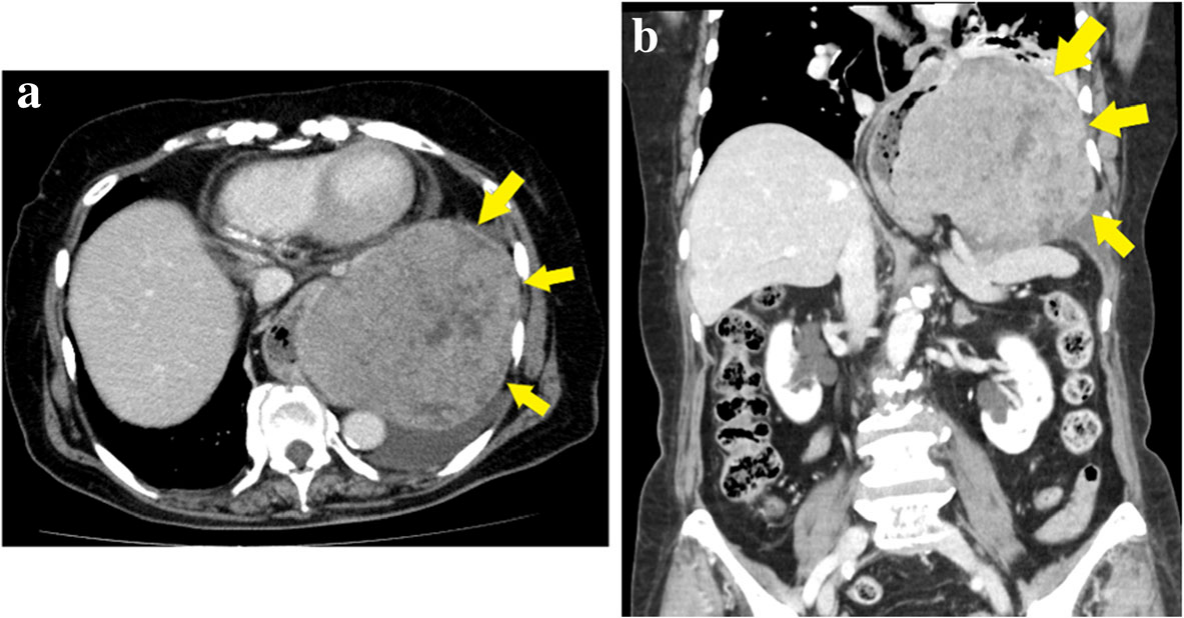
Chest and abdominal computed tomographic scans showing a 13-cm mass in the mediastinum. a Axial view. b Coronal view
